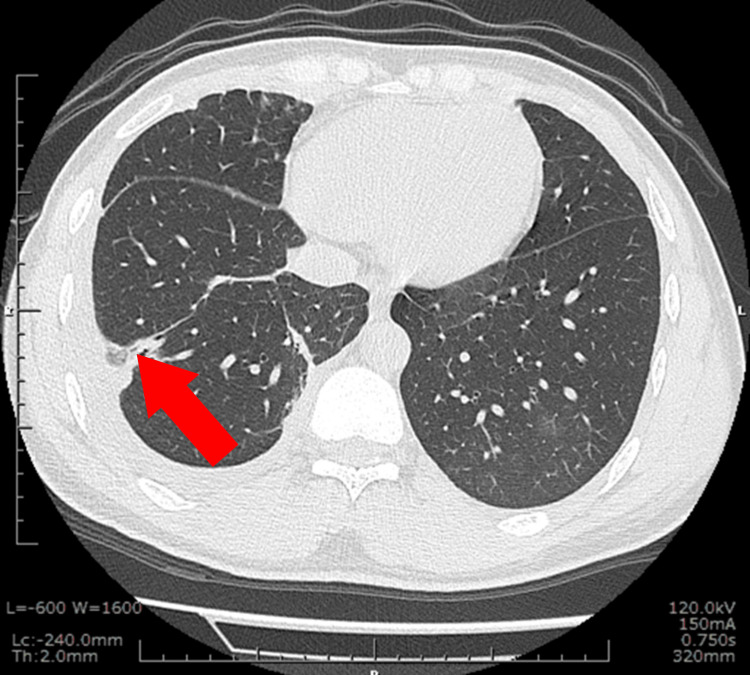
Non-contrast chest CT image Non-contrast chest CT shows an infiltrative shadow in the right lower lobe, with a cavity-like lesion suggestive of a migration tract. The red arrow indicates a subpleural linear opacity abutting the visceral pleura, a finding suggestive but not pathognomonic of paragonimiasis.
